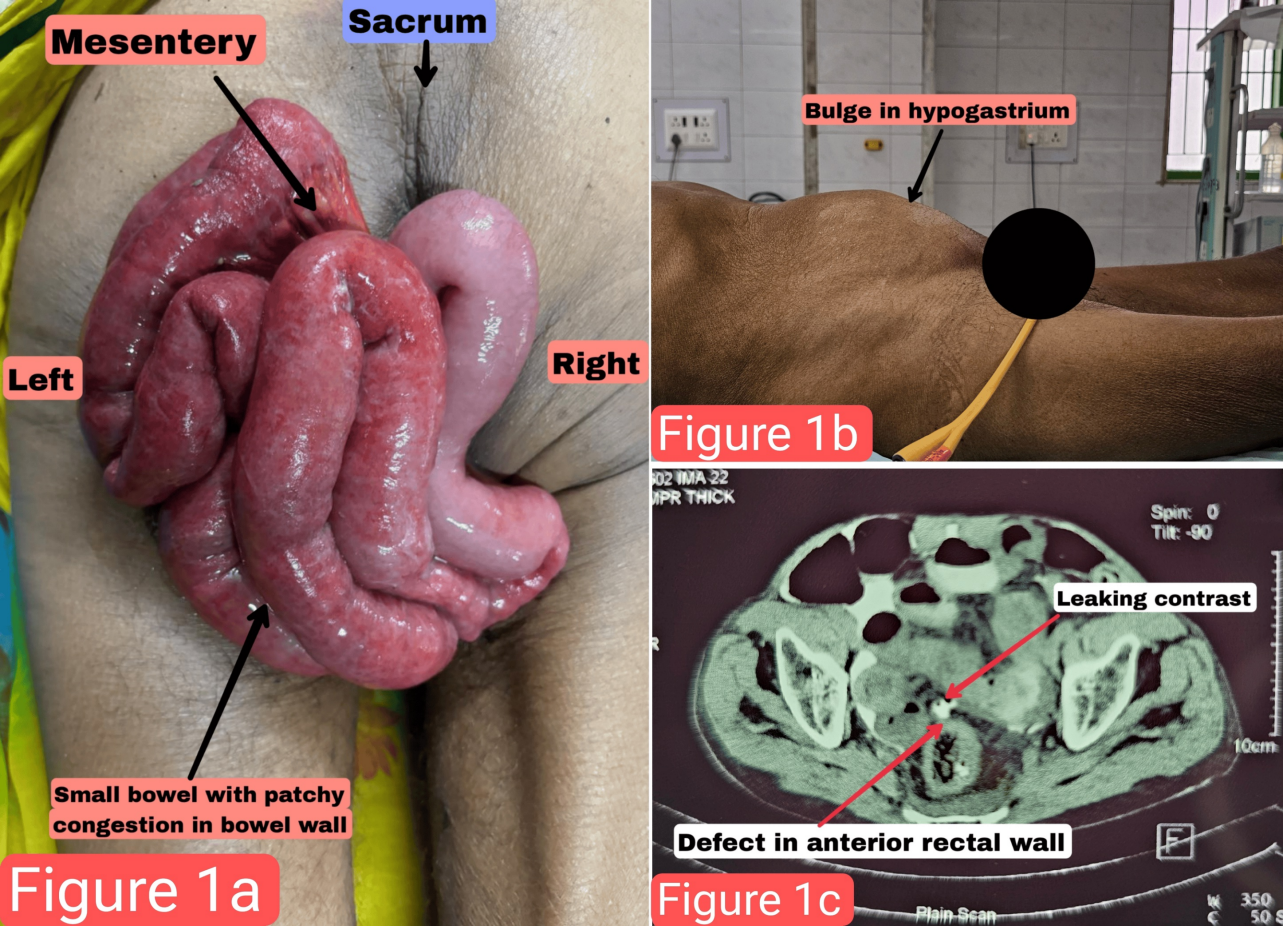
(a) Prolapsed small bowel. (b) Bulge in the hypogastrium. (c) Contrast-enhanced CT scan of the abdomen showing perforation on the anterior aspect of the upper rectal wall.
